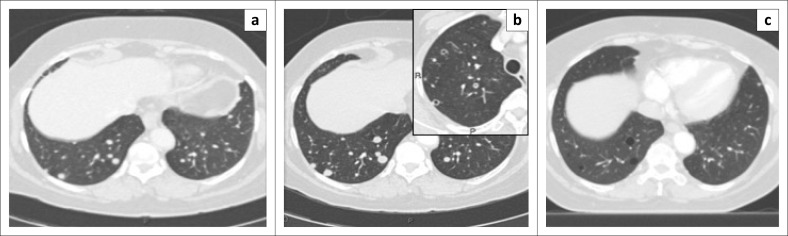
Consecutive computed tomography axial image series through the lung bases eloquently depicts the temporal evolution of: (a) solid pulmonary nodules to (c) thin-walled cysts. The magnified insert clearly demonstrates the central cavitation (b).
